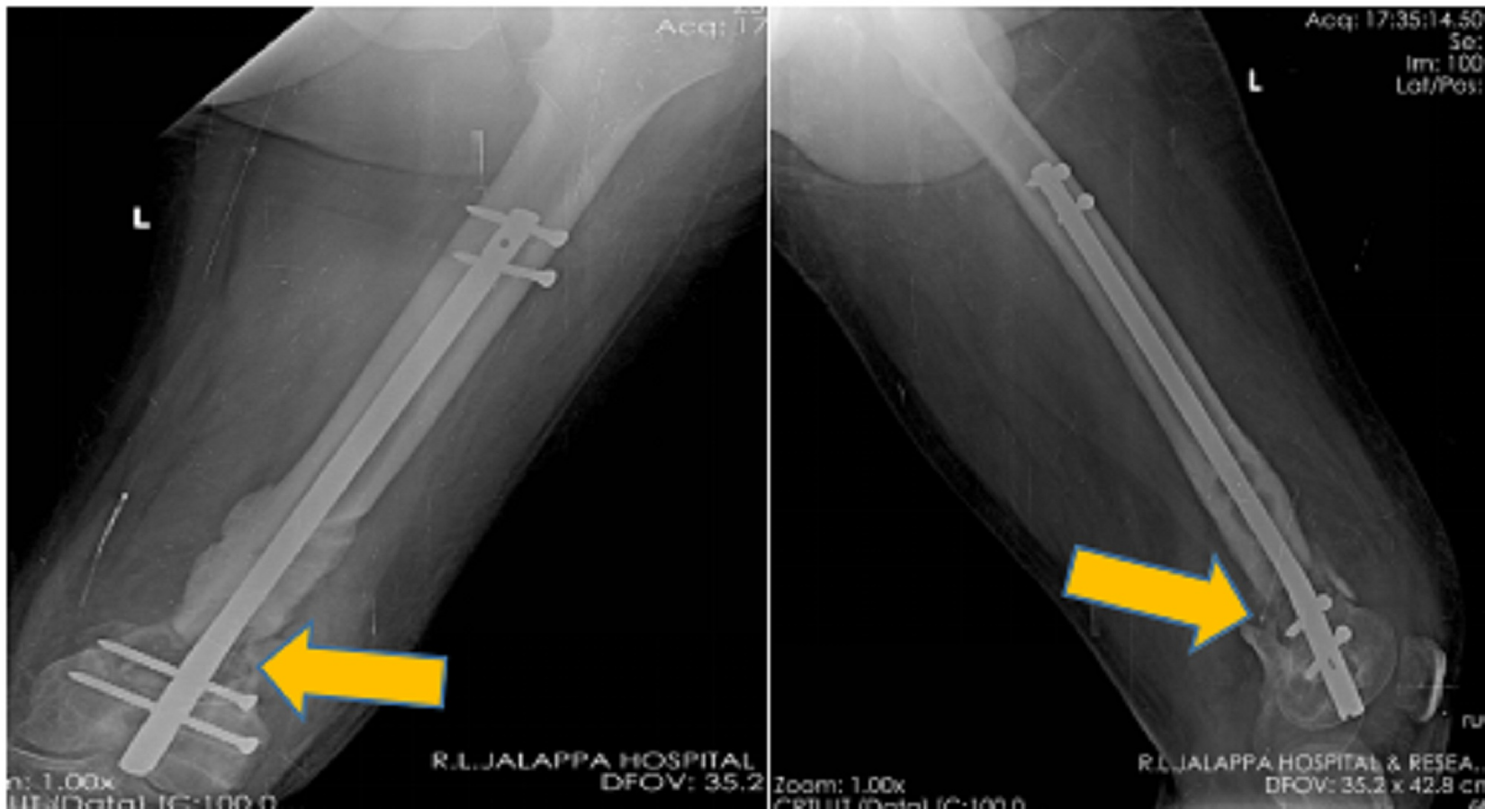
Post-operative left thigh antero-posterior and lateral view radiograph showing removal of the locking compression plate with distal femoral nail fixation with allogenic bone grafting.
